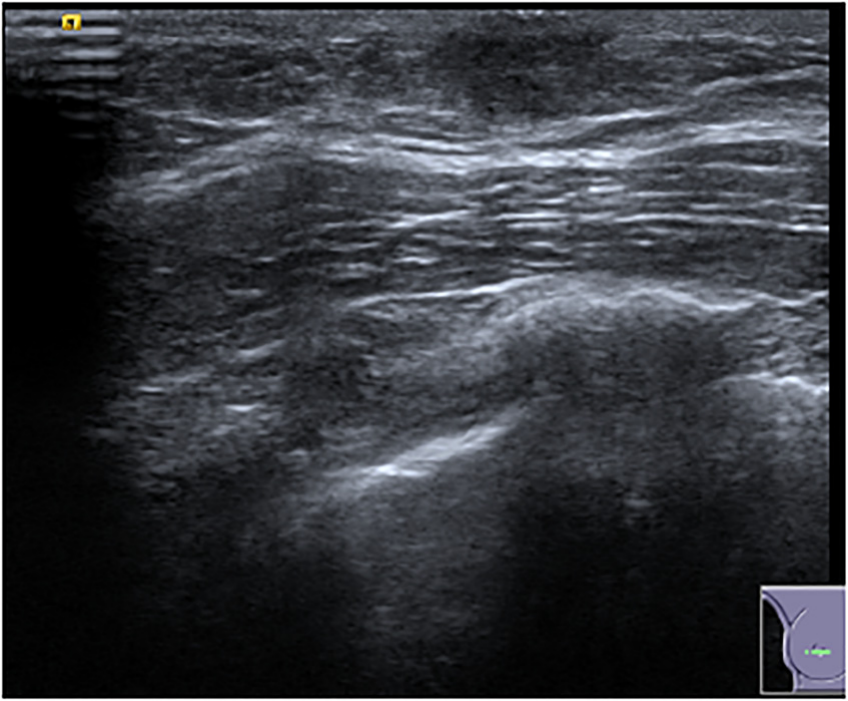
B-mode ultrasound shows retroareolar triangular, hypoechoic disc-shaped nodule in keeping with gynecomastia.
